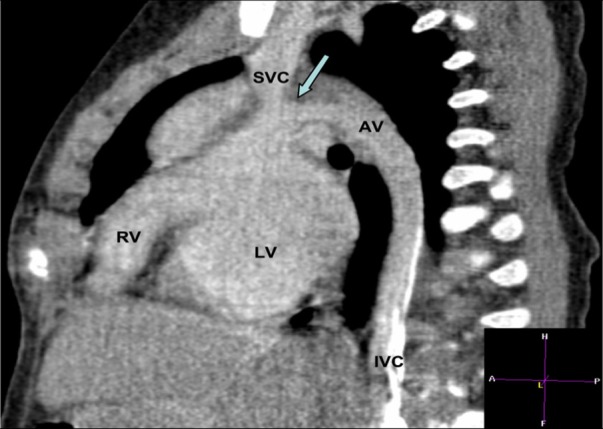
CT angiography scan showing the continuation of the interrupted inferior vena cava into the azygos vein with a narrow connection (arrow) in a patient with hypoplastic right ventricle. AV, azygos vein; IVC, inferior vena cava; LV, left ventricle; RV, right ventricle; SVC, superior vena cava.
